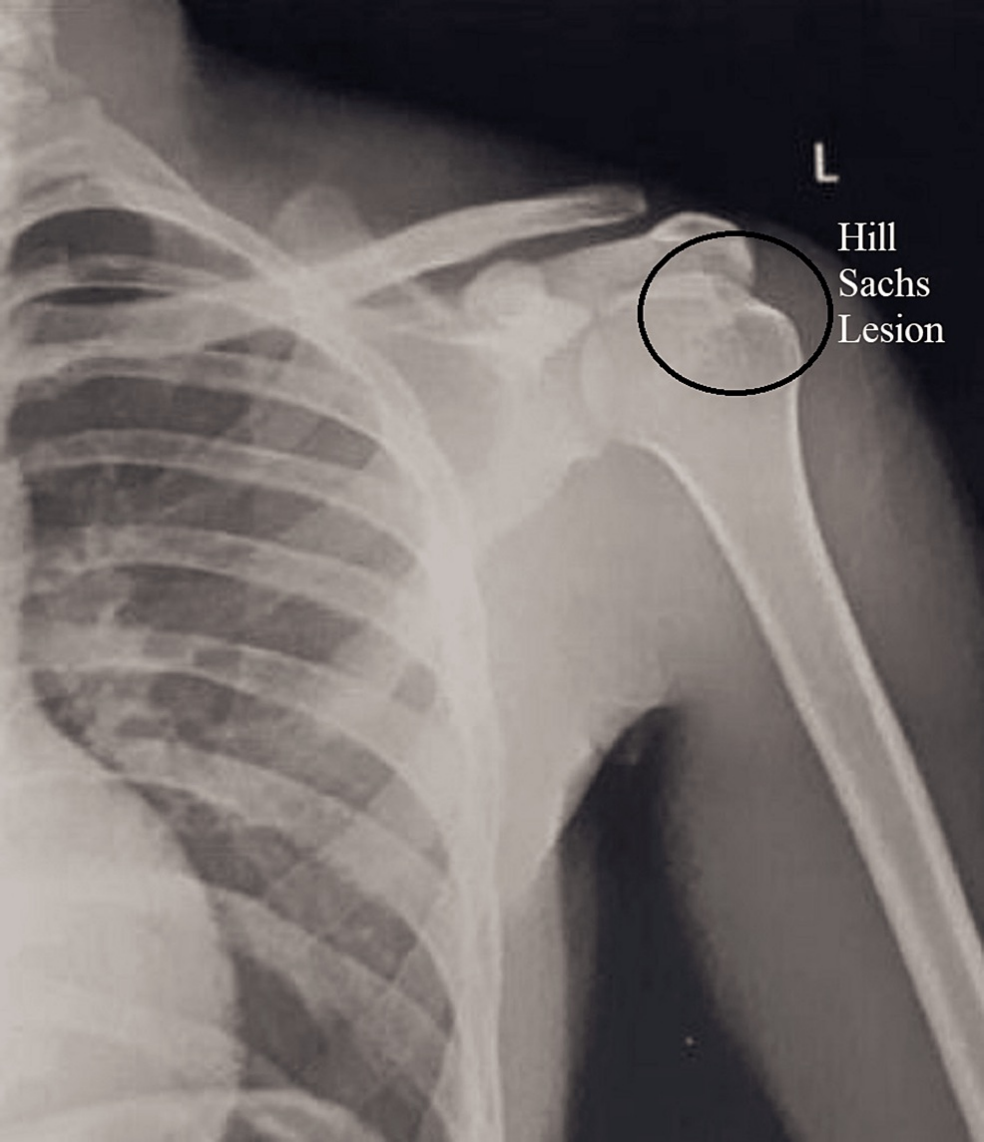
X-ray of the left shoulder
